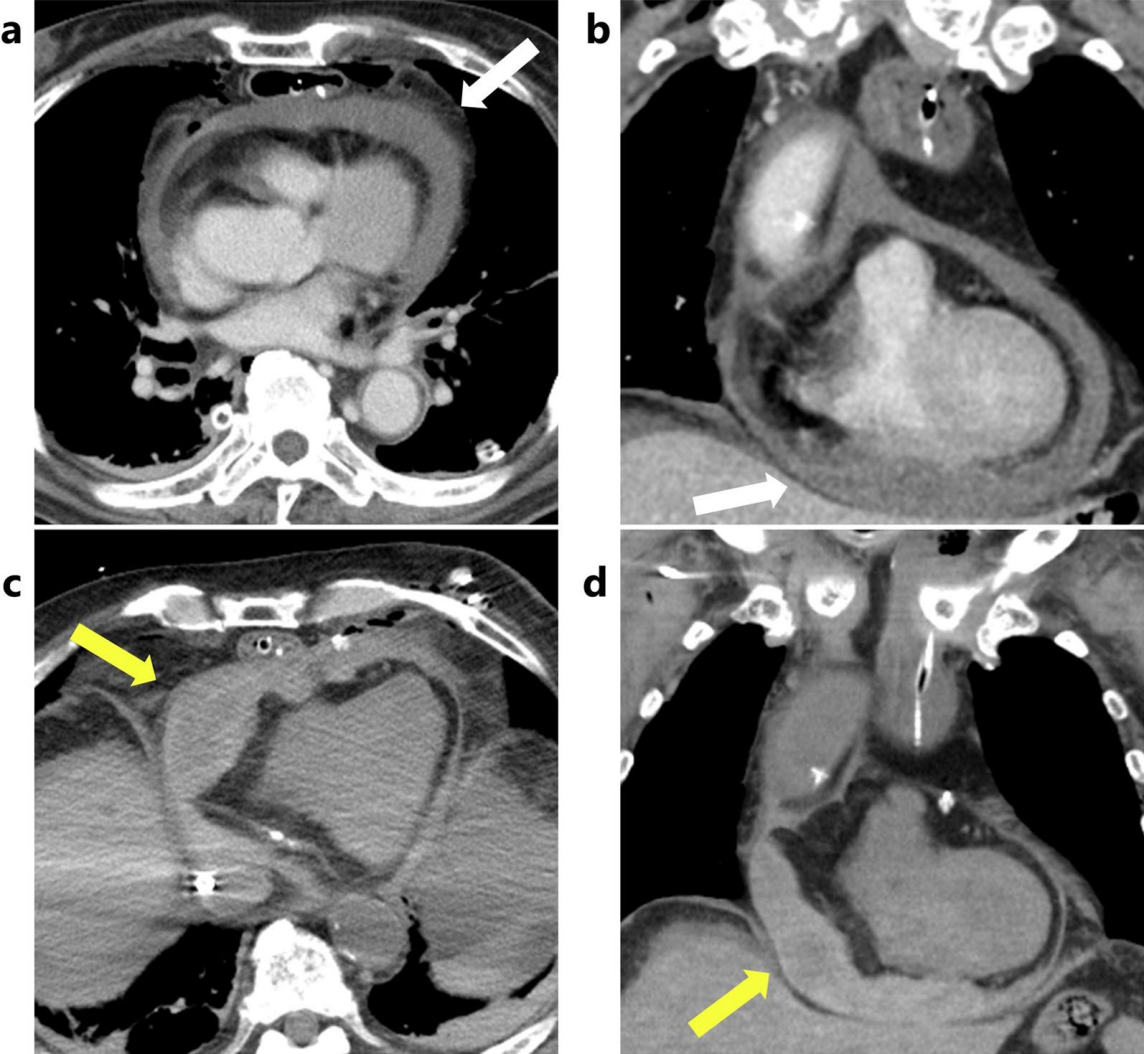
Computed tomography images. a, b Images before the first drainage showed a high-density fluid accumulation in the pericardium (white arrows). c, d Images before the second drainage showed an enlarged residual hematoma around the right ventricle (yellow arrows)
